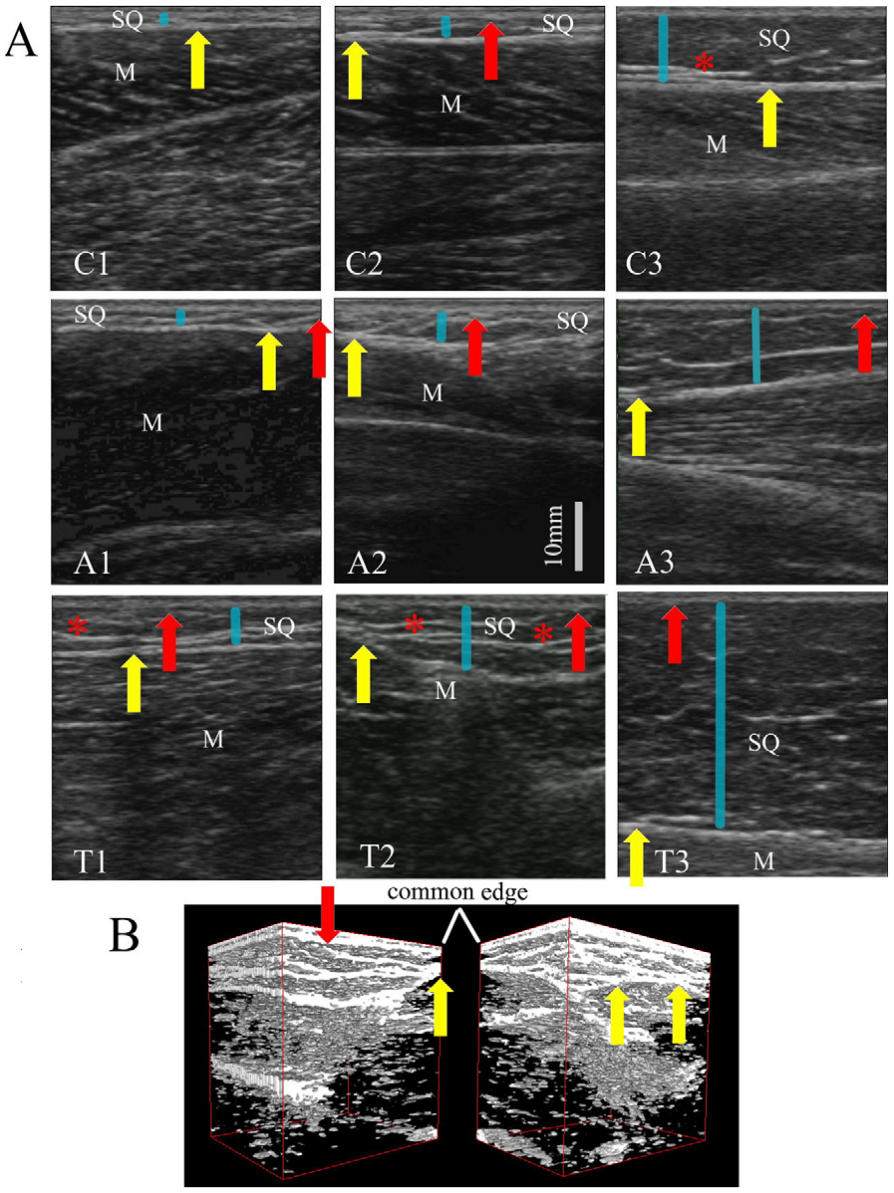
Ultrasound images showing the morphological characteristics of fascial bands. (A). Ultrasound images from calf, arm and thigh; (B). Three dimensional reconstruction of a video sweep, visualized from two different perspective angles.
